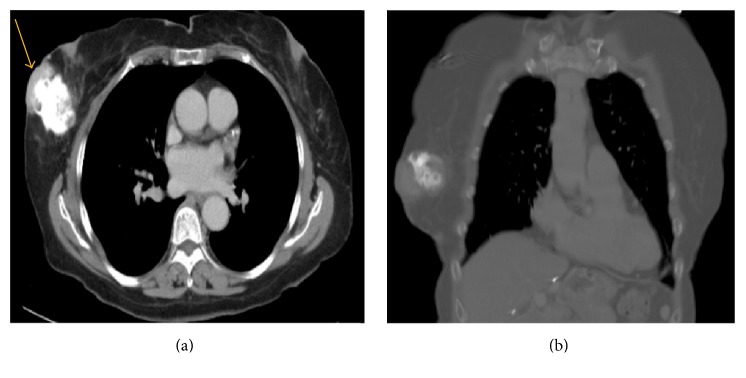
Axial (a) and coronal (b) contrast-enhanced CT to characterize the lesion and rule out metastasis. A heavily calcified right breast mass was noted measuring about 3.2 × 5.8 cm with overlying skin thickening.
